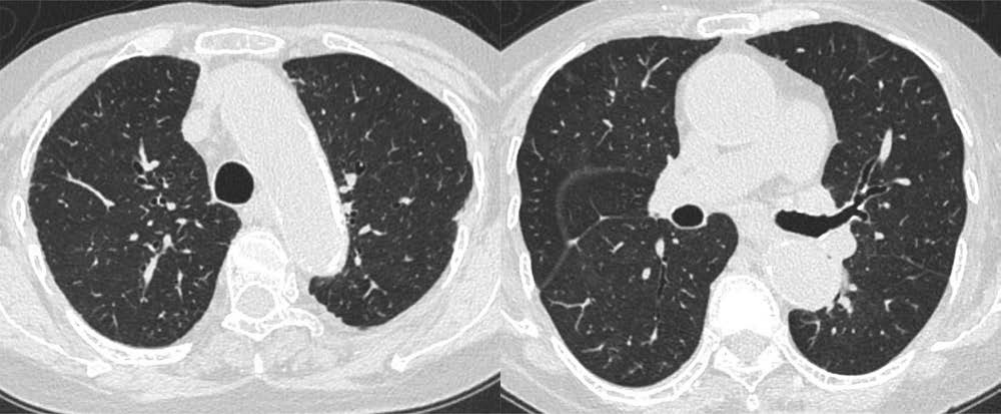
A chest computed tomography image after the steroid treatment.
